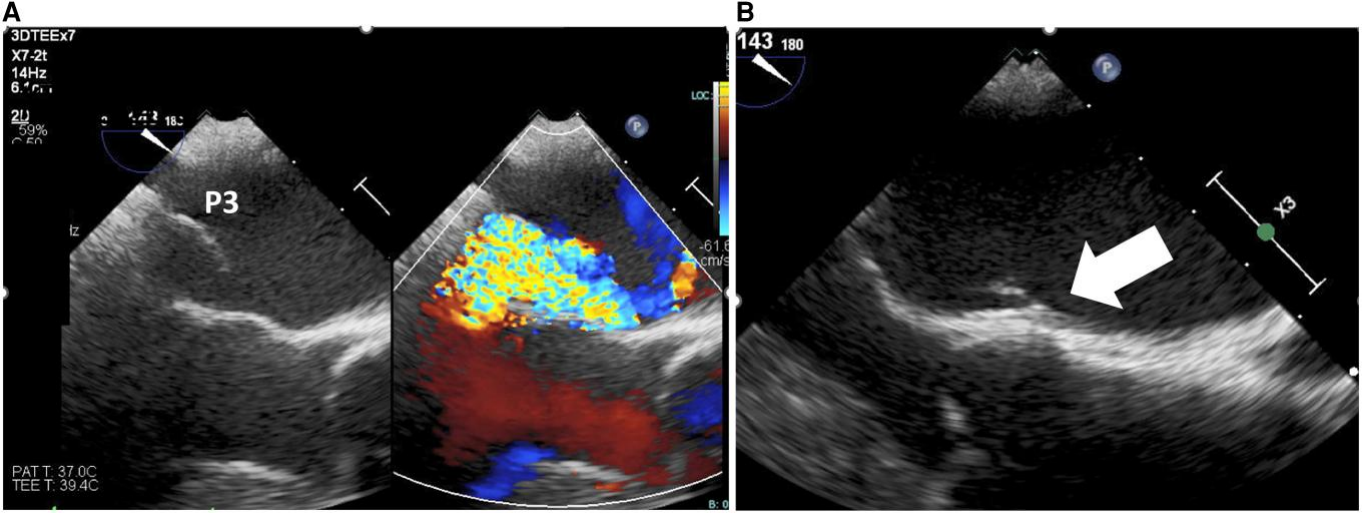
First transoesophageal echocardiography. (*A*) Mid-oesophageal aortic valve long-axis view showed mitral regurgitation due to P3 prolapse. (*B*) Magnified image of the same view showed vegetation on atrial septum where the mitral regurgitation jet impinged (arrow).
